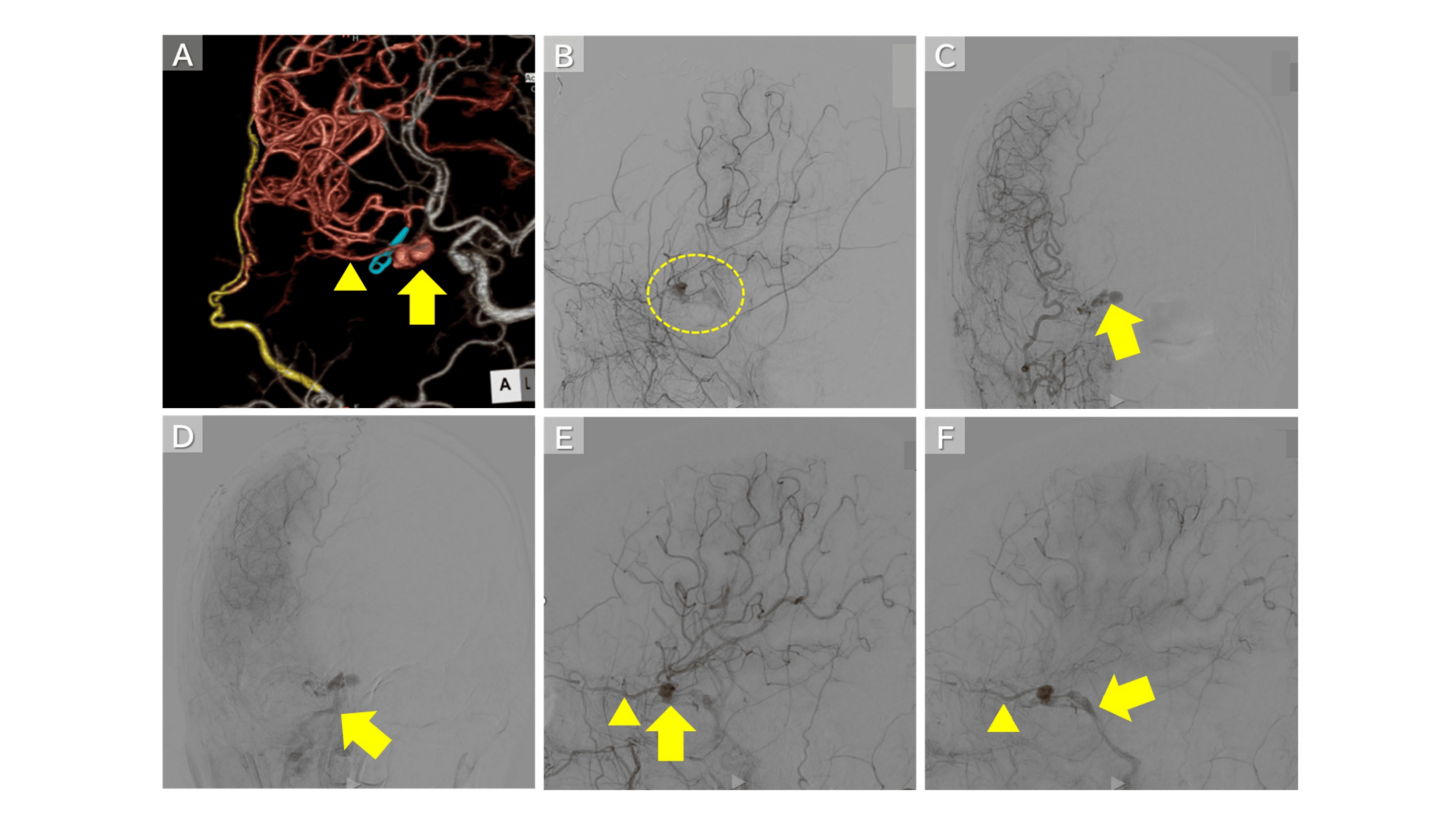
Imaging Findings After Double STA-MCA Bypass and ICA Trapping (A) CTA on postoperative day 4 showed suspected flow from the ophthalmic artery (arrowhead) into the pseudoaneurysm (arrow). (B) Cerebral angiography demonstrated retrograde filling of the pseudoaneurysm and findings suggestive of a CCF (circle). (C, E) Arterial-phase imaging revealed retrograde flow from the ophthalmic artery (arrowhead) into the pseudoaneurysm (arrow). (D, F) Venous-phase imaging showed a CCF draining into the inferior petrosal sinus (IPS) (arrow). Arrowhead indicates the ophthalmic artery. STA-MCA: superficial temporal artery-middle cerebral artery; ICA: internal carotid artery; CTA: computed tomography angiography; CCF: carotid-cavernous fistula
